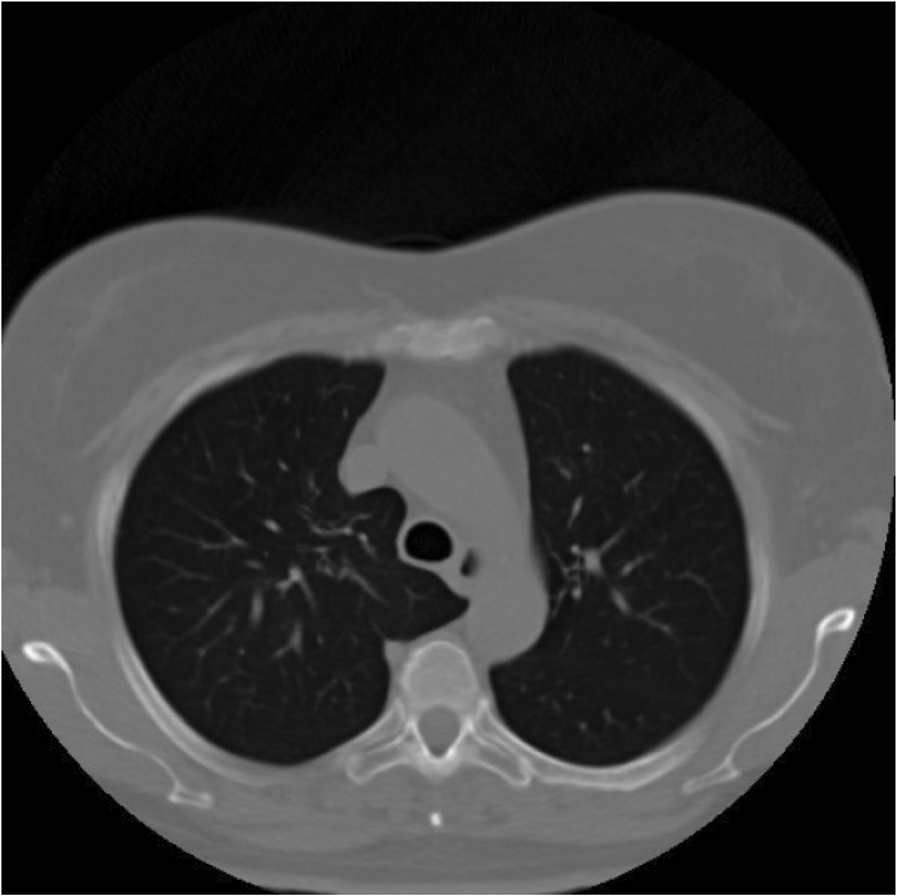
Chest CT scan after treatment with everolimus.
